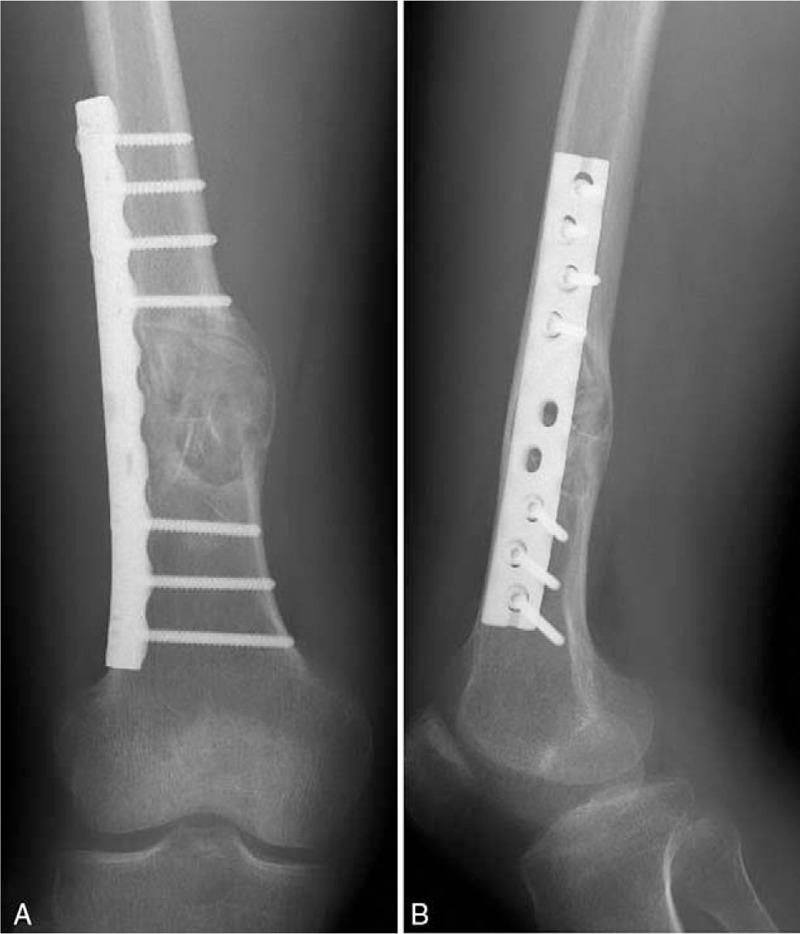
Post-operation radiography of the right femur. After lesion curettage, bone graft and steel plate fixation were performed.
